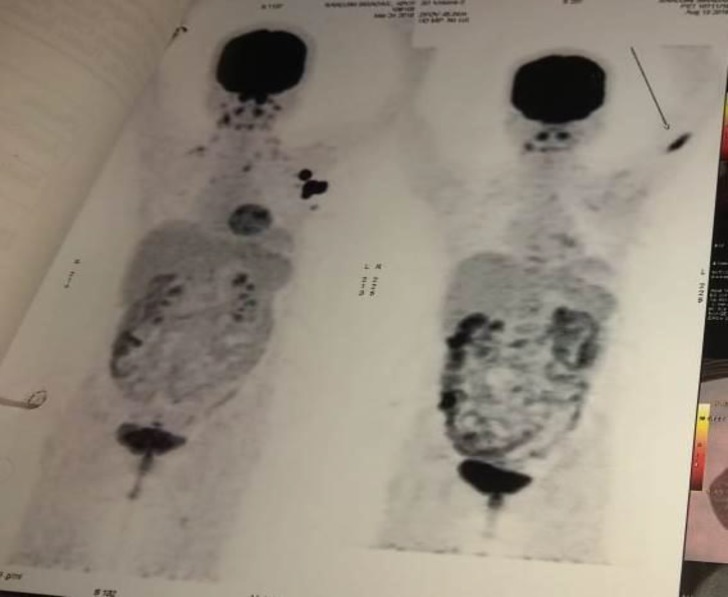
Showing Positron Emission Tomography- Computed Tomograohy (PET-CT) image of the patient with hyper metabolic left axillary lymph nodes.
